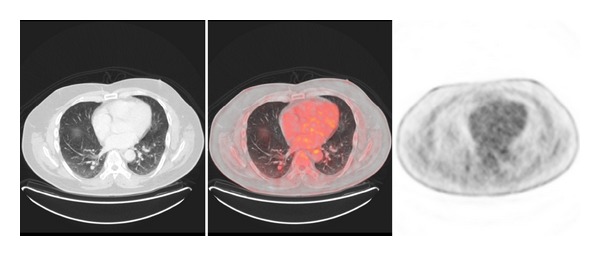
Axial view with pulmonary node in right lower lobe of 1.1 cm and SUVmax 2.5 suggestive of metastasis.
